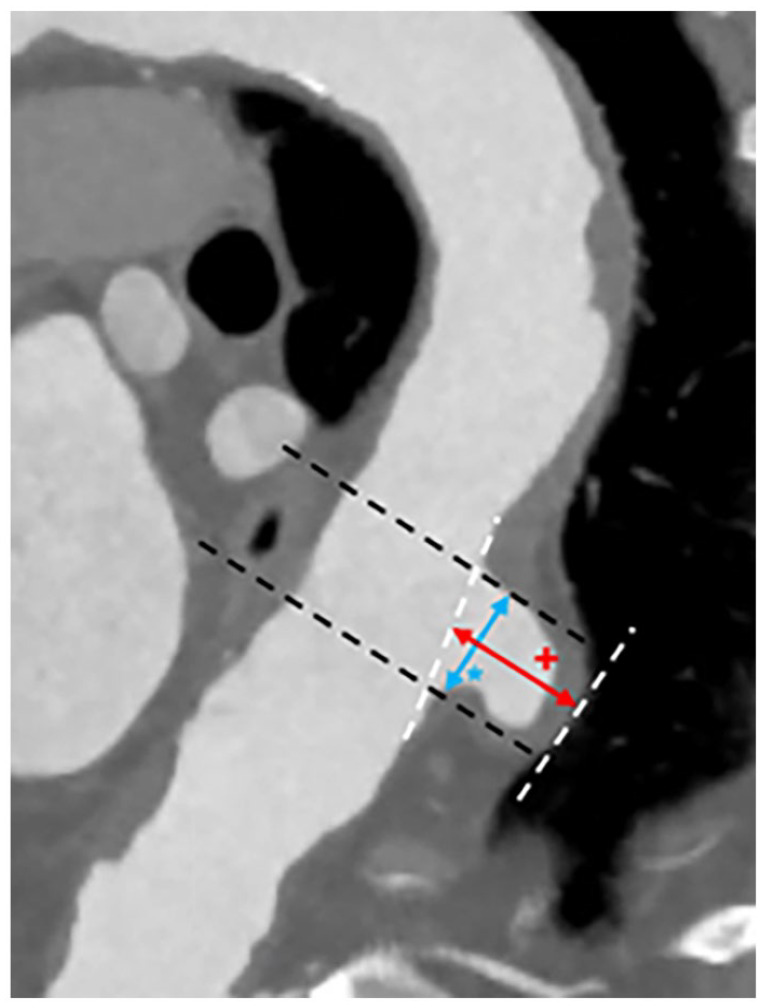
PAU measurement (CTA): PAU depth (red +), PAU wide (blue*).
